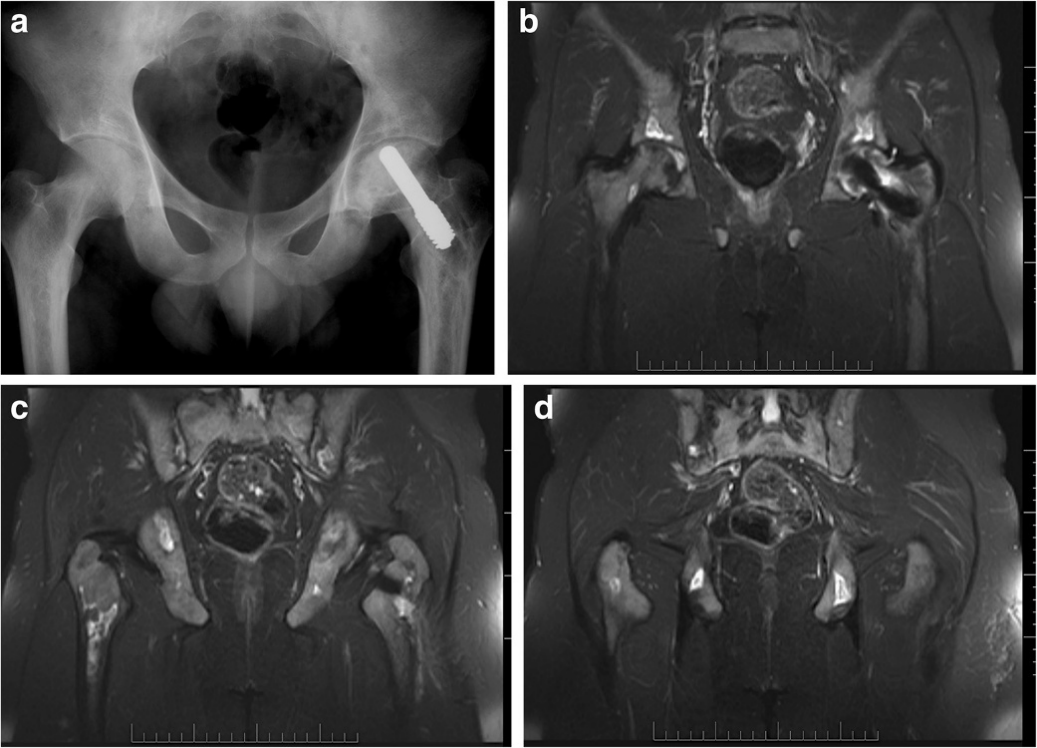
A 23-year-old patient with a history of hemoglobinopathy (B-thallasaemia) and osteonecrosis of the left hip, which was treated with porous tantalium rod implantation. The patient presented at the follow up examination with multiple painful sites in the pelvis. Plain AP x-ray of the pelvis was negative (a). The coronal fat suppressed T2-w TSE images (b-d) depicted multiple bone infarcts bilaterally at the ischiac bones, acetabuli and proximal femoral diaphyses.
